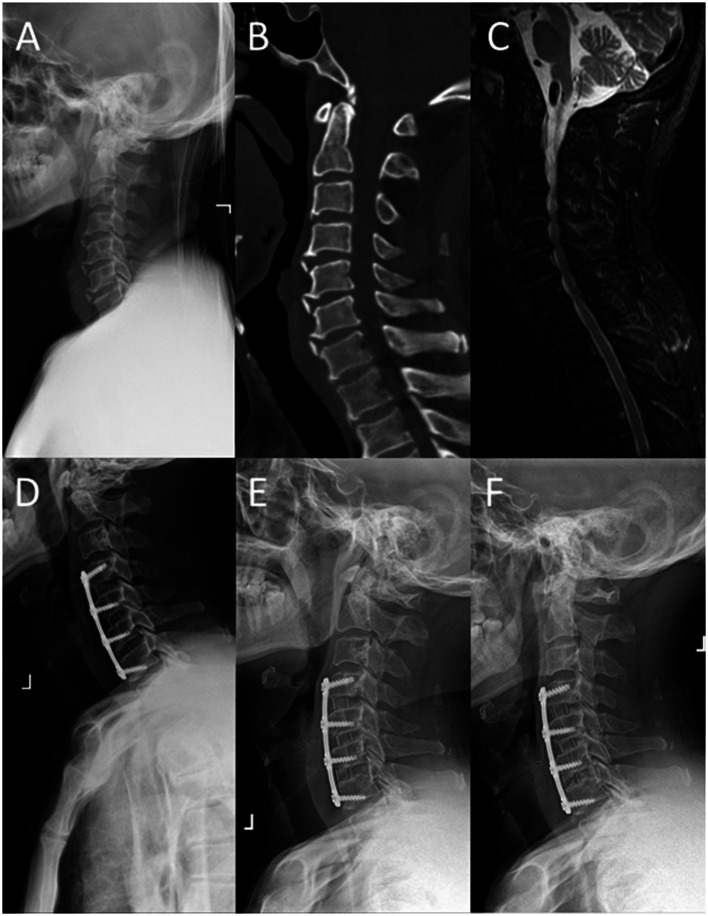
A 52-year-old male was treated with 3-level ACDF. (A) X-ray lateral view before surgery; (B) sagittal CT scan before surgery; (C) sagittal fat-suppressed sequence in MRI before surgery; (D) postoperative X-ray lateral view; (E) X-ray lateral view at 1 month after surgery; and (F) X-ray lateral view at FFU.
